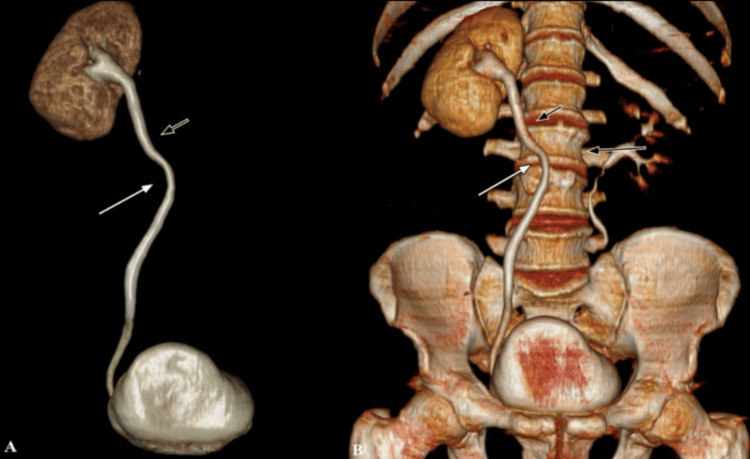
CTU with 3D coronal VR Coronal VR in the delayed phase shows the medial deviation of the right mid ureter (at the level of L3 vertebra) with an “S” shaped deformity of the right proximal ureter. Note: Right proximal ureter (short black arrow), Right mid ureter (long white arrow), L3 vertebra (long black arrow). CTU: Computed tomography urography, 3D: Three-dimensional, VR: Volume rendered.
